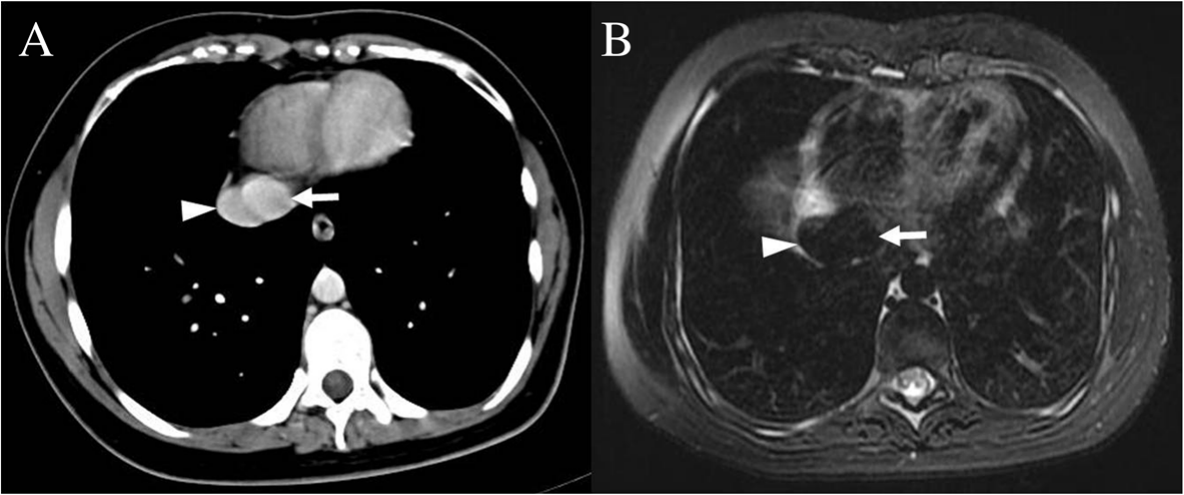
Abdominal precontrast computed tomography (CT) and magnetic resonance imaging (MRI). The portal trunk drains directly into the inferior vena cava (IVC) just below the level of the right atrium, shown on both postcontrast computed tomography (CT) (A) and T2-weighted magnetic resonance imaging (MRI) (B). White arrowhead = IVC; white arrow = portal trunk.
